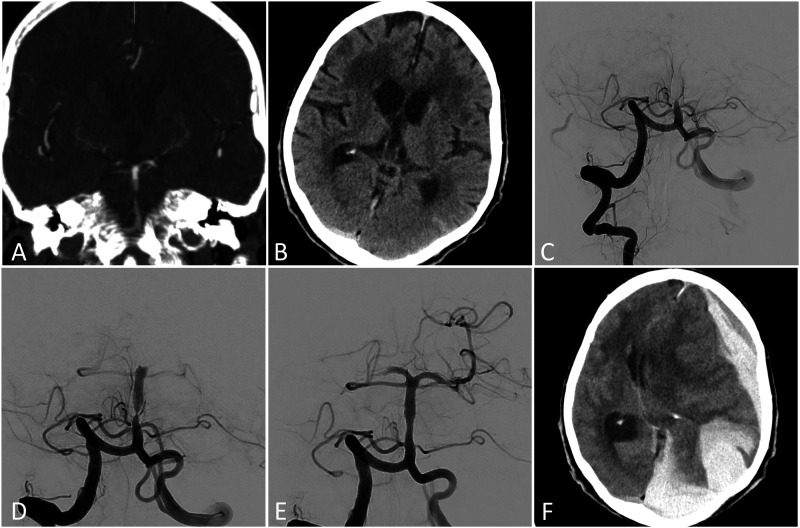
An 80-year-old female patient with acute basilar artery occlusion, treated with intravenous thrombolysis at the referring centre (not shown). Upon arrival computed tomography showed a persistent proximal basilar artery thrombosis with reperfusion of the basilar apex (a). Native computed tomography demonstrated a slight subarachnoid haemorrhage in the left temporal region (b). The first angiogram showed persisting occlusion of the proximal third of the basilar artery (c). After one aspiration the vessel was recanalised, but revealed a proximal high-grade stenosis (d), which re-occluded 10 min later. The implantation of a self-expanding stent with following percutaneous transluminal angioplasty led to good reconstitution of the vessel (e). Recanalization of the occluded right P2 segment was not attempted. As the patient was already pretreated with a daily dose of 100 mg of aspirin, an intravenous infusion of tirofiban was initiated with the intention to load the patient with clopidogrel in an overlapping fashion. Four hours later the patient demonstrated wide and fixed pupils bilaterally. An emergency computed tomography scan showed a massive haemorrhage with intraparenchymal, subarachnoid and subdural components. Surgical evacuation was not attempted, and the patient died 2 days later.
